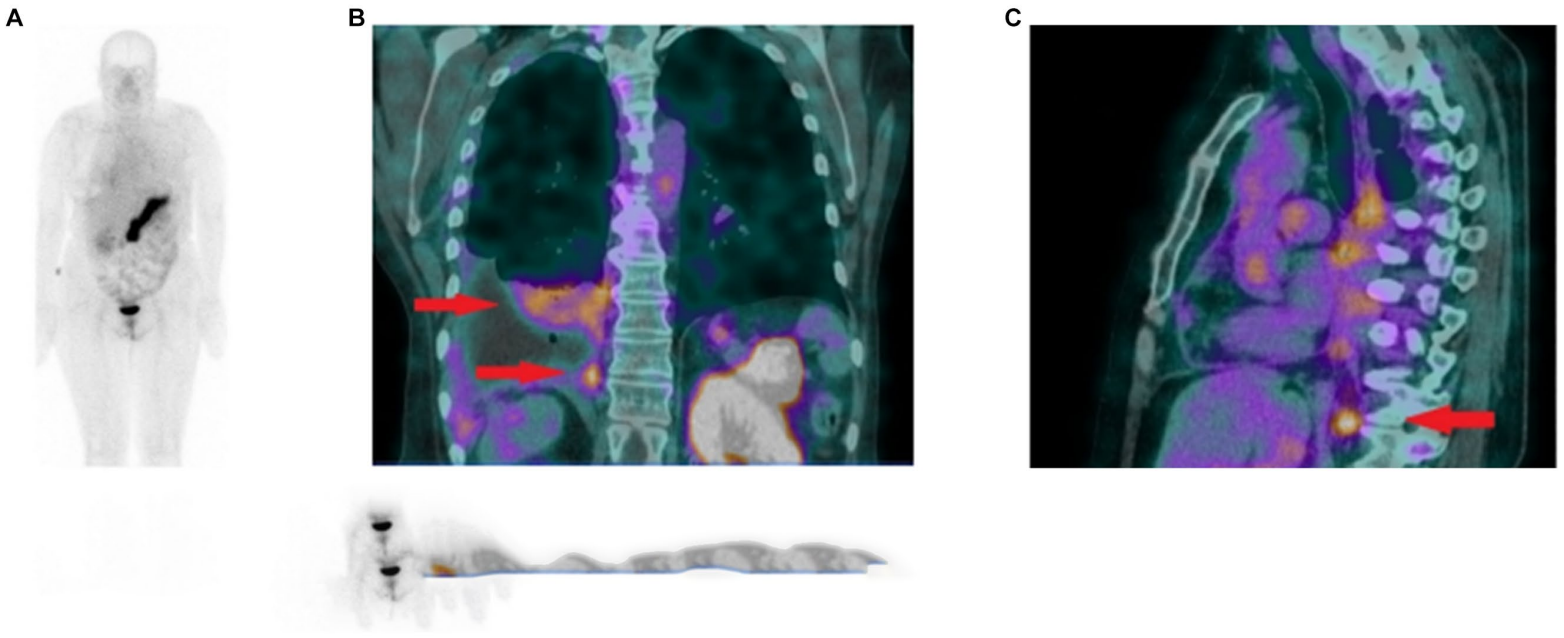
(A) Whole body scan obtained 3 h after injection of [^99m^Tc]Tc-DB15 in the anterior projection shows physiological accumulation in the pancreas and increased uptake in the right pleura. (B) SPECT/CT coronal image of the chest presenting increased tracer uptake in the metastatic infiltrations in the pleura and lung parenchyma (red arrows). (C) SPECT/CT sagittal image of the chest depicting increased radioactivity accumulation in an enlarged (metastatic) phrenic lymph node (red arrow). Adapted from [99mTc]Tc-DB15 in GRPR-Targeted Tumor Imaging with SPECT: From Preclinical Evaluation to the First Clinical Outcomes by Nock et al. (90), under Creative Commons Attribution (CC BY) license.
